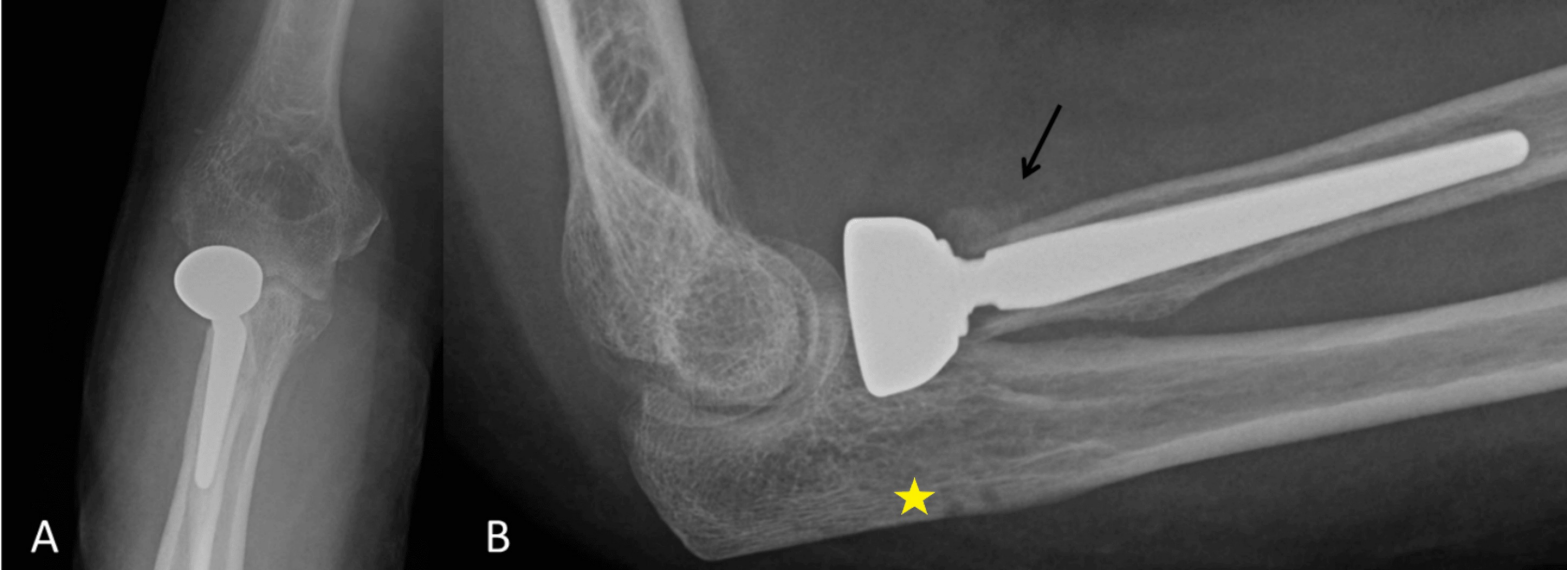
Post-operative radiographs at four weeks after surgery. A: Antero-posterior view demonstrating a well-fitting long-stemmed RHR. B: Lateral view demonstrating the appearance of callus in the lateral radial neck (black arrow), at the site of the fracture that was noted intra-operatively. Also, note the transosseous drill holes in the ulna that were used to repair the coronoid tip fracture (yellow asterisk). RHR, radial head replacement
